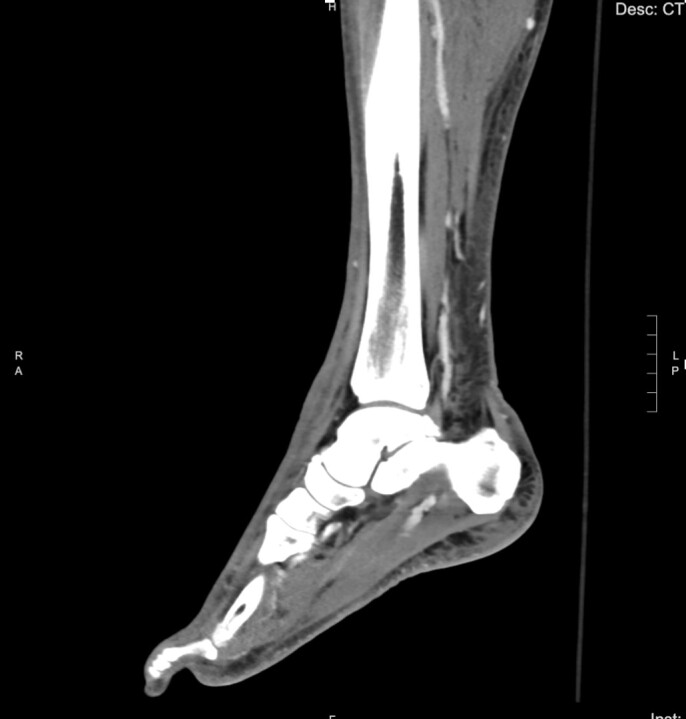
Computed tomography sagittal view of the distal right lower extremity of a 45-year-old male with lower extremity wounds, swelling, and a rash.
